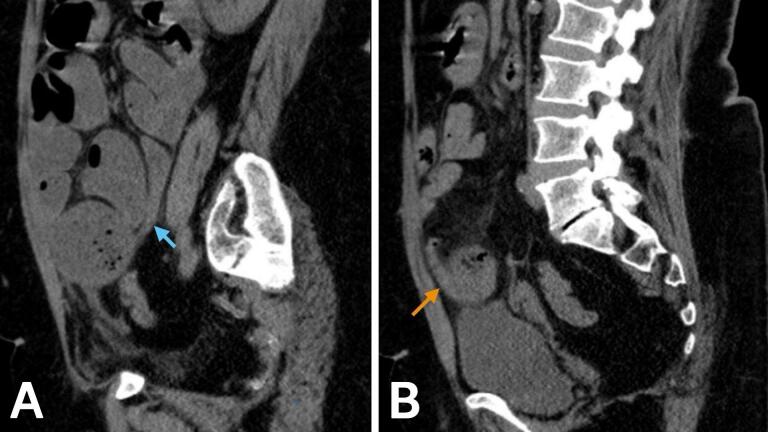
Sagittal abdominal CT without IV contrast. Both images A and B show tapering involving both ends of the closed loop, representing a double beak sign. The proximal and distal limbs are indicated by the blue and orange arrows, respectively. (For interpretation of the references to colour in this figure legend, the reader is referred to the web version of this article.)
